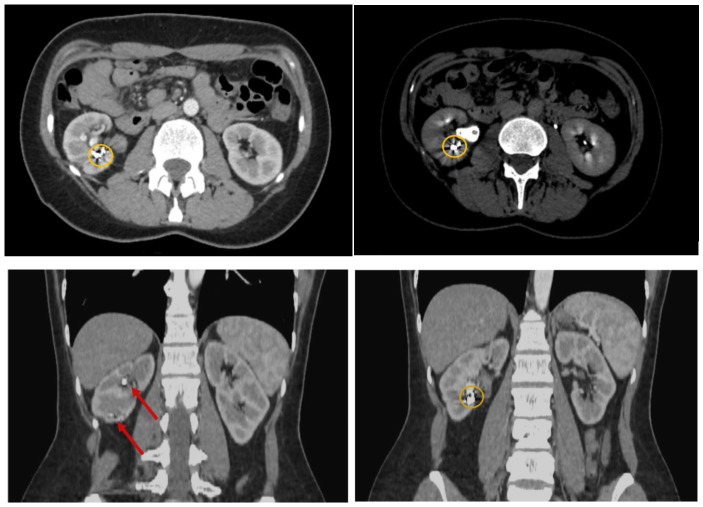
Contrast-enhanced CT (axial and sagittal views) revealing multiple hematic accumulations at various stages of degradation (indicated by orange circles) and lithiasis (indicated by red arrows).
